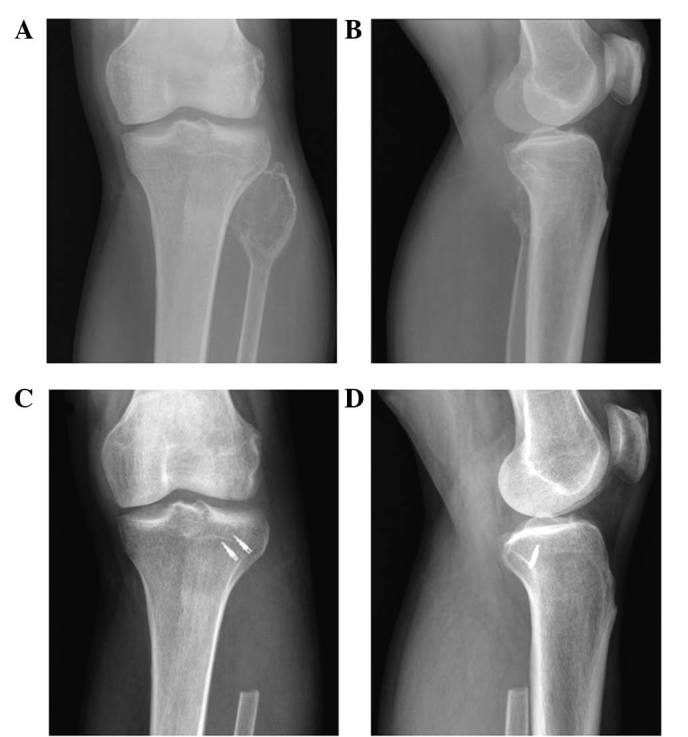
(A and B) Preoperative plain radiograph shows a 35-year-old male with a giant cell tumor of the proximal fibula. (C and D) Postoperative plain radiograph shows the lateral collateral ligament and biceps femoris tendon reattached to the lateral tibial metaphysis.
